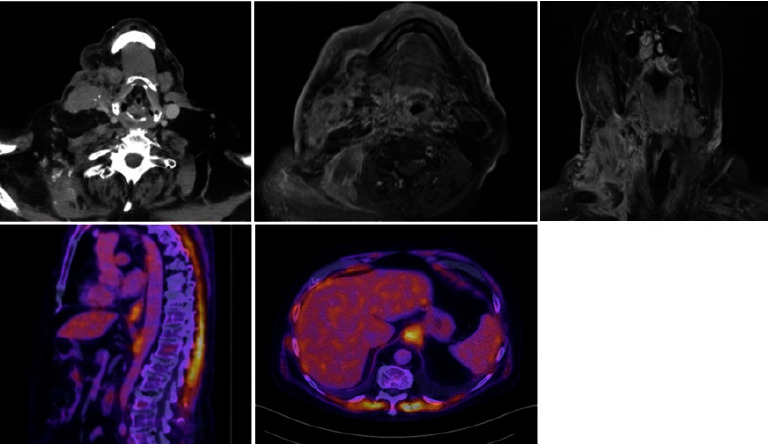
PET/CT scan and MRI of the patient. PET/CT scanning was performed following the intravenous administration of F-18 FDG and intravenous and enteric contrast. F-18 emission and CT attenuation acquisitions were performed, utilizing diagnostic-quality CT. CT FINDINGS: no masses or areas of abnormal enhancement are identified in the head and neck region. No lymphadenopathy is identified. PET FINDINGS: there is hypermetabolic activity in the lower esophagus and GE junction. While this is likely physiologic or inflammatory activity, malignancy is not excluded. MRI results demonstrate extensive expansile infiltrative process representing known metastatic disease involving the right neck and shoulder musculature, particularly the sternocleidomastoid, anterior scalene, and levator scapulae.
